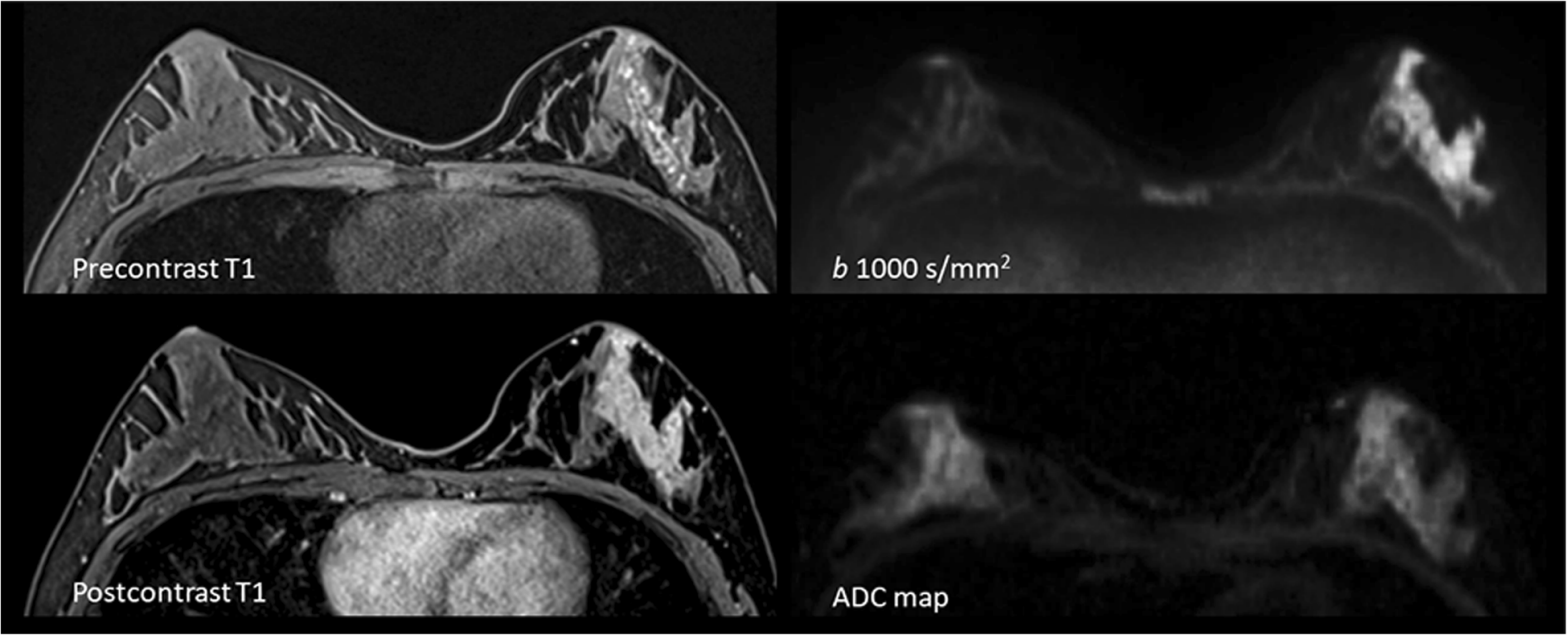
A 23-year-old patient with invasive ductal carcinoma. Hemorrhagic ductal content is seen at the lateral part of the left breast on precontrast T1. There is a segmental enhancement pattern on postcontrast T1. Diffusion restriction is detected on DW-MRI and ADC map. Transverse plane provides a synchronized evaluation
